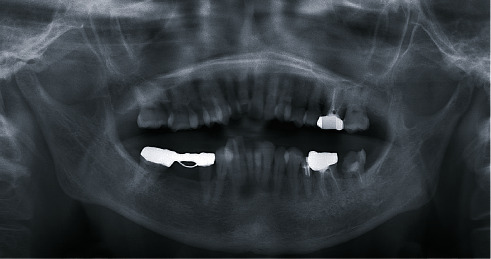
Panoramic radiograph from the first examination. The left mandibular condyle is flattened, and radiopaque images are seen near the superior joint cavity.
